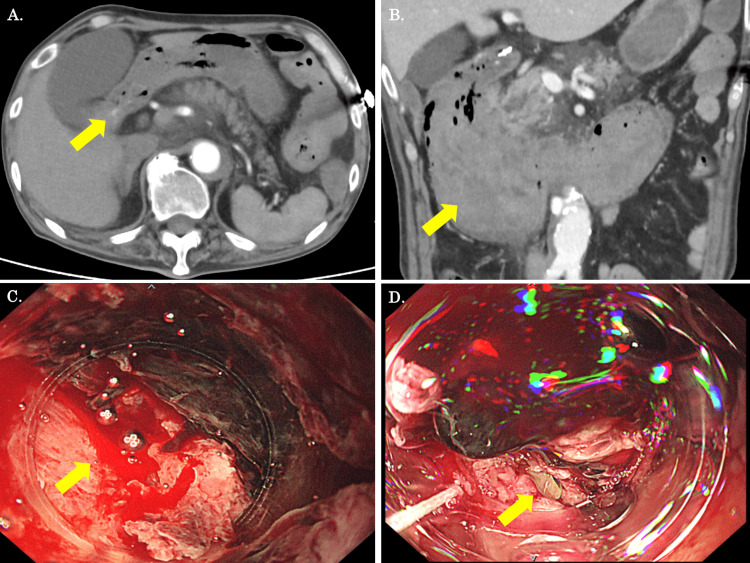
CT and EGD findings prior to the emergency operation (A, B) An enhanced CT scan showed that extravasation was present around the duodenal clipping point, and the duodenum was significantly dilated due to massive hematoma (yellow arrow). (C) An EGD showed that active bleeding was observed at the posterior lateral aspect of the duodenum (yellow arrow). (D) An EGD showed a mucosal defect that appeared to be perforation after OTSC (yellow arrow). EGD, esophagogastroduodenoscopy; OTSC, over-the-scope clip
